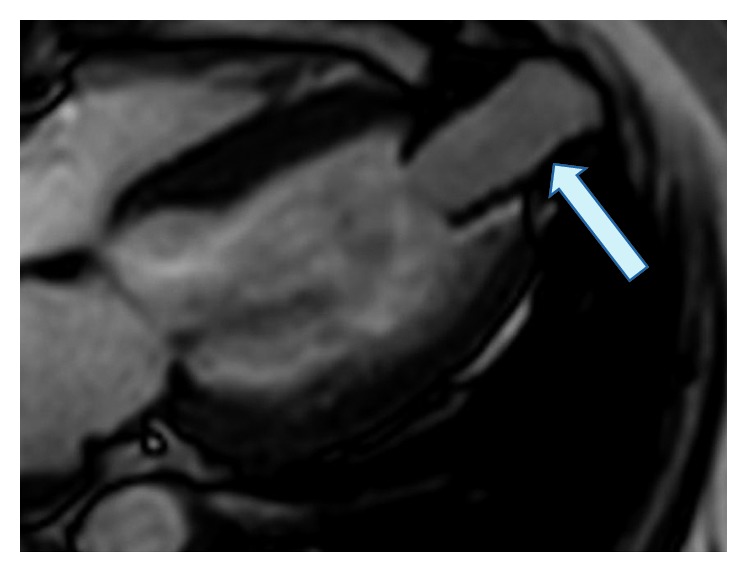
MRI shows a conduit from the apex of the left ventricle (arrow).
